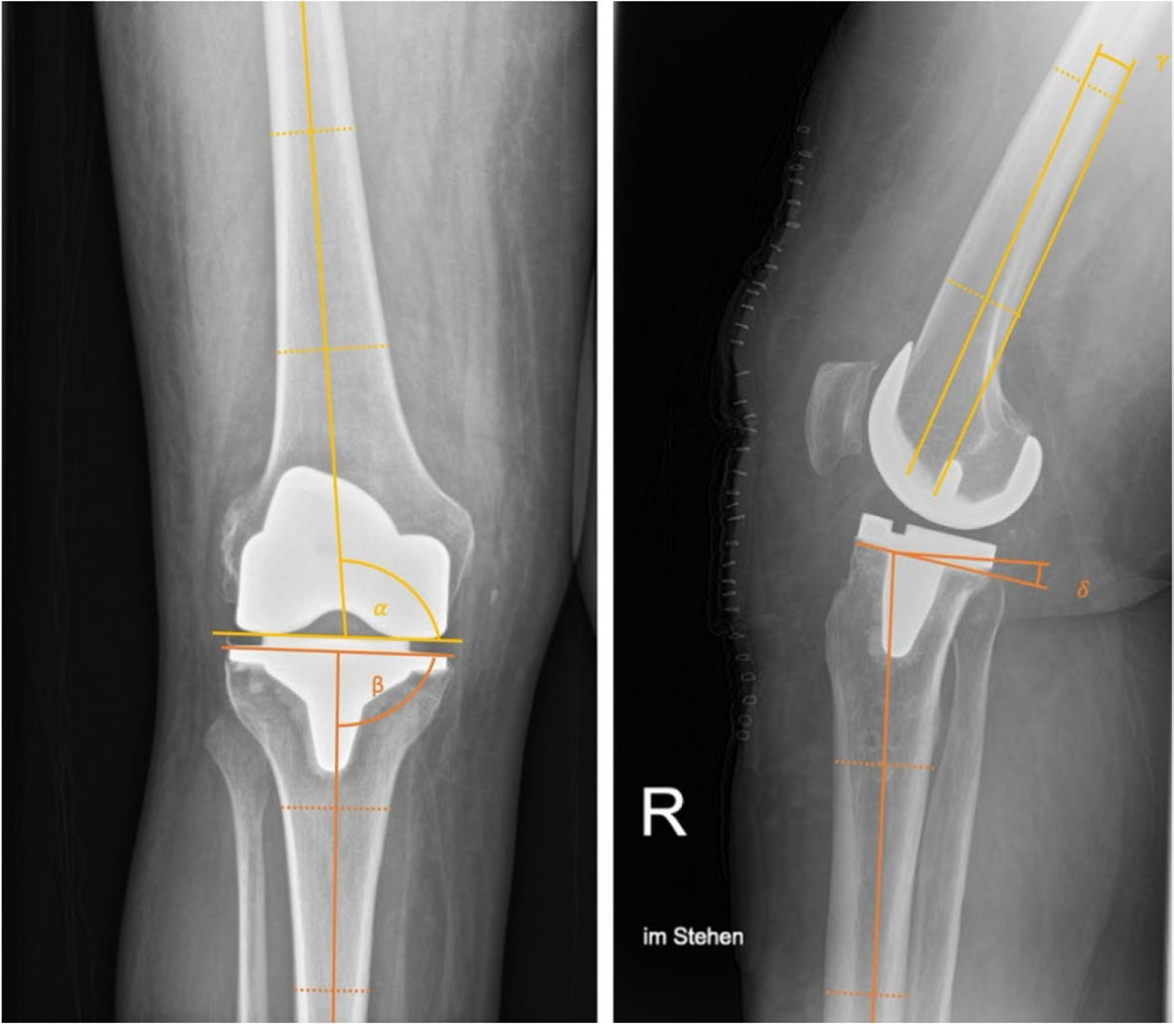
Measurement of component alignment. Representative images demonstrating the measurement of component alignment parameters on postoperative radiographs of a right knee. The Distal Femoral Angle (DFA, α) was defined as the medial angle between the femoral anatomical axis and the distal surface of the femoral component (left image). The Proximal Tibial Angle (PTA, β) was measured as the medial angle between the tibial anatomical axis and a line parallel to the tibial baseplate (left image). The Sagittal Femoral Angle (SFA, γ) was defined as the angle between the most distal femoral fixation surface in relation to the femoral shaft axis (right image). The Posterior Slope Angle (PSA, δ) was defined as the angle formed between the tibial anatomical axis and the tibial baseplate (right image)
